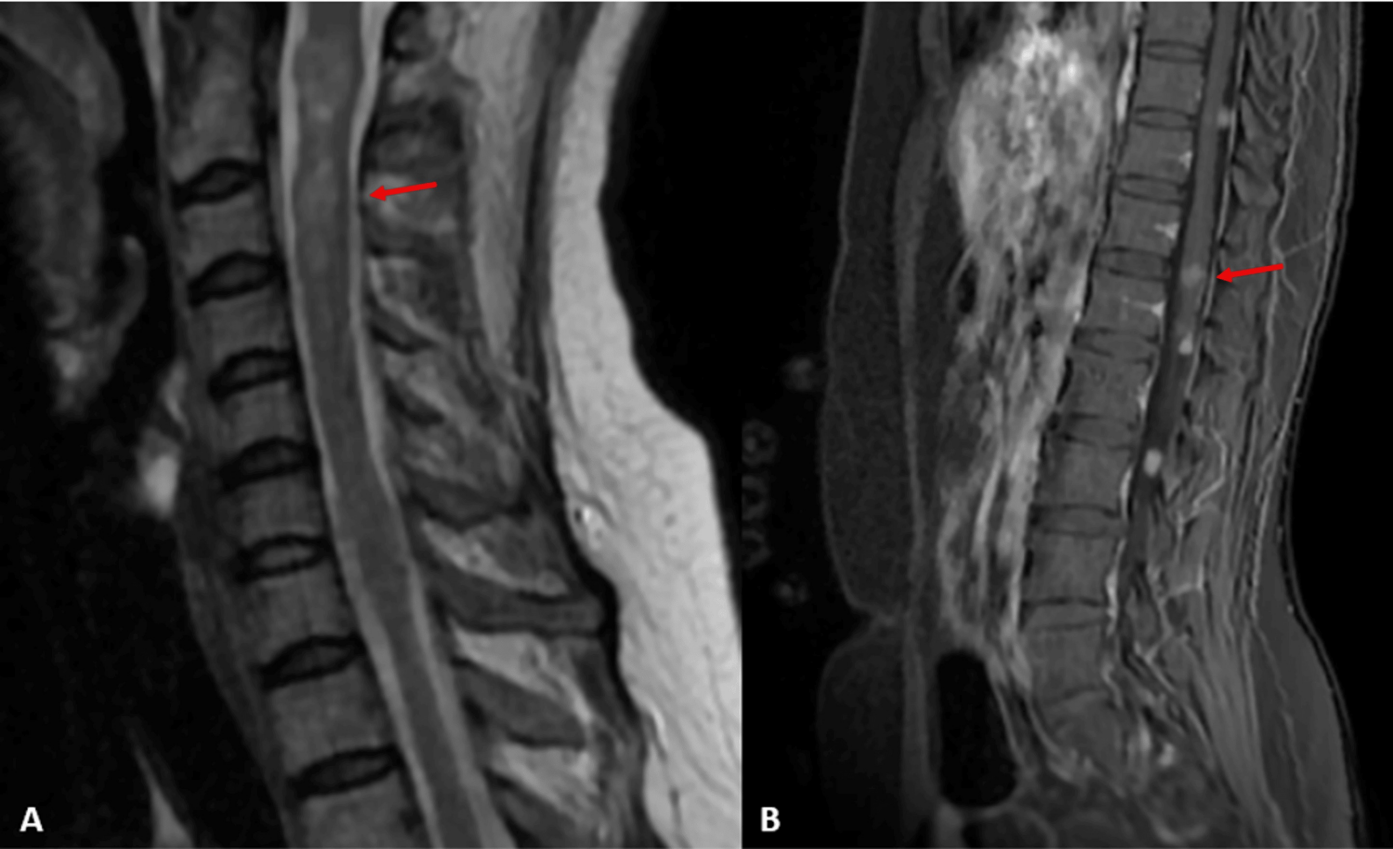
(A) MRI of the spinal cord sagittal section, T2 sequence, objectifying cervicodorsal spinal cord astrocytoma and meningiomas. (B) MRI of the spinal cord sagittal section, T1 sequence injected, demonstrating dorsolumbar spinal cord astrocytoma and meningiomas.
